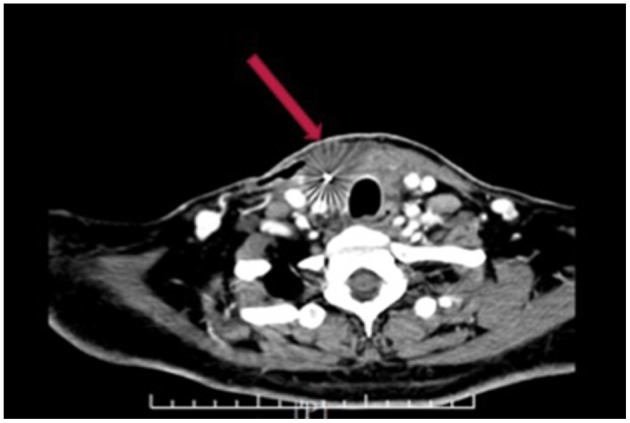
Cervical enhanced CT 1 month after first ^125^I seed implantation.
